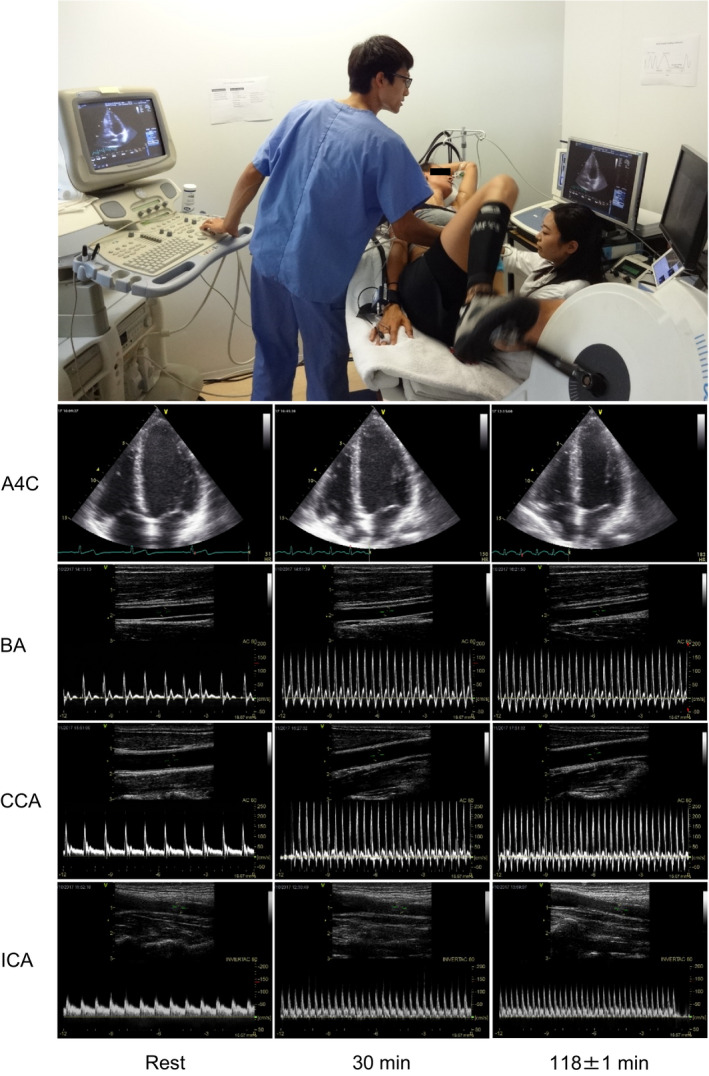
Examples of echocardiographic and Doppler ultrasonographic assessments during prolonged submaximal semi‐recumbent cycling. The photo depicts one of the participants in the study. Representative images of apical 4‐chamber view at end diastole (A4C) and vessel and blood velocity recordings at the brachial artery (BA), common carotid artery (CCA), and internal carotid artery (ICA), at rest and during exercise (30 and 118 ± 1 min) in the progressive dehydration trial are shown
